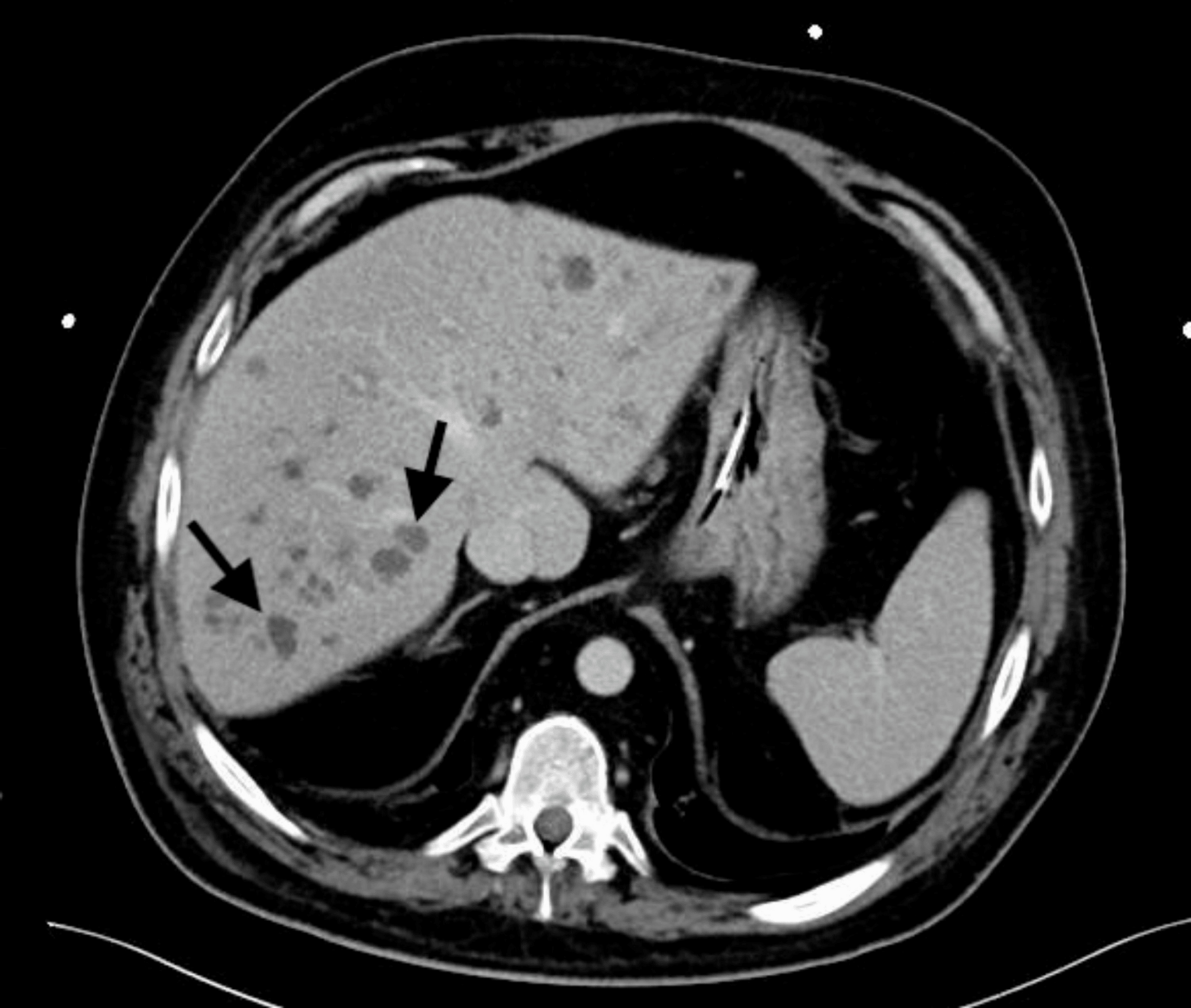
Abdominal CT scan showing multiple hepatic hypodense lesions (arrows) CT: computed tomography
